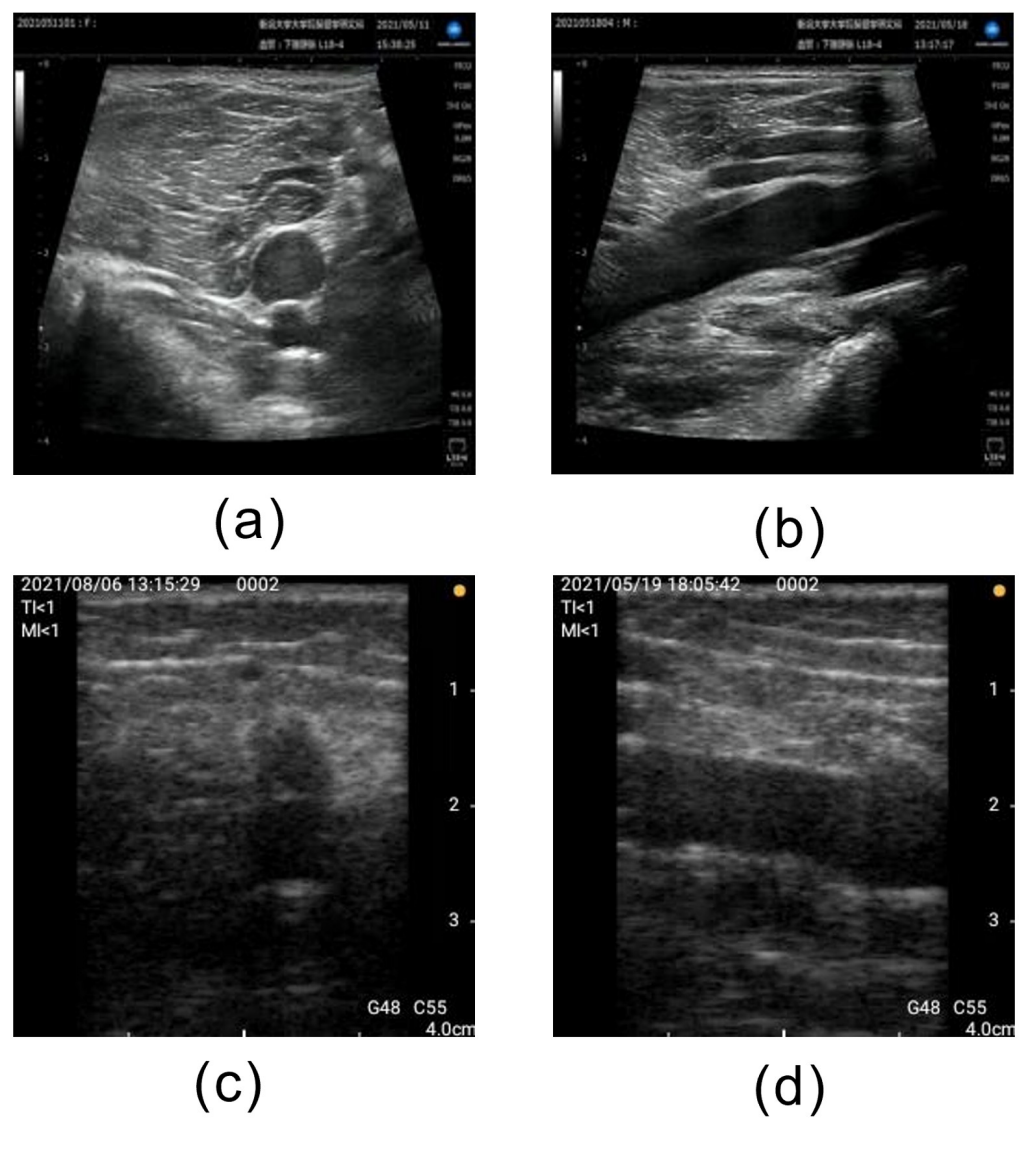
Examples of correctly classified satisfactory images. (a) Short axis acquired by stationary ultrasonography equipment, (b) Long axis acquired by stationary ultrasonography equipment, (c) Short axis acquired by portable ultrasonography equipment, and (d) Long axis acquired by portable ultrasonography equipment.
